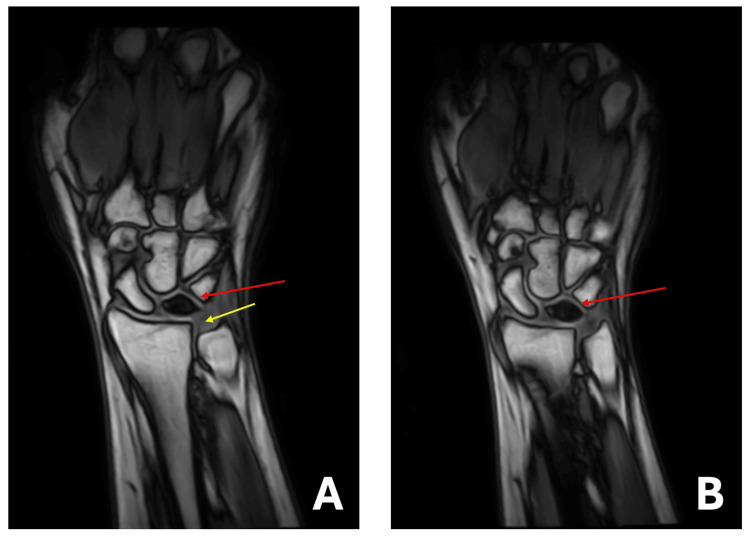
(A, B) Multiplanar multisequence MRI of T1-weighted images of the left wrist. The yellow line indicates negative ulnar variance, while the red lines show decreased signal intensity representative of lunate osteonecrosis. MRI: magnetic resonance imaging.
